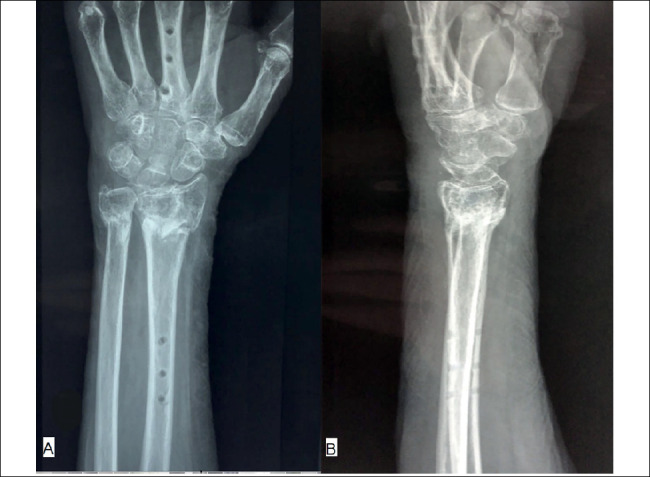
Radiographic images in AP (A) and lateral (B), two months postoperatively, after removing the spanning plate.
